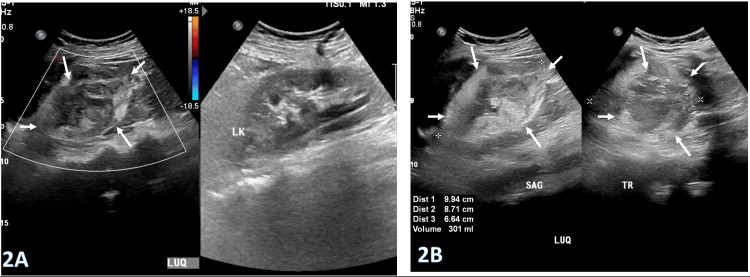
Selected ultrasound images (2A and 2B) demonstrate a large heterogeneous lesion in the left upper quadrant (LUQ) measuring 9.9 × 8.7 × 6.6 cm (arrows; approximate volume: 300 mL), with no significant internal color flow.
